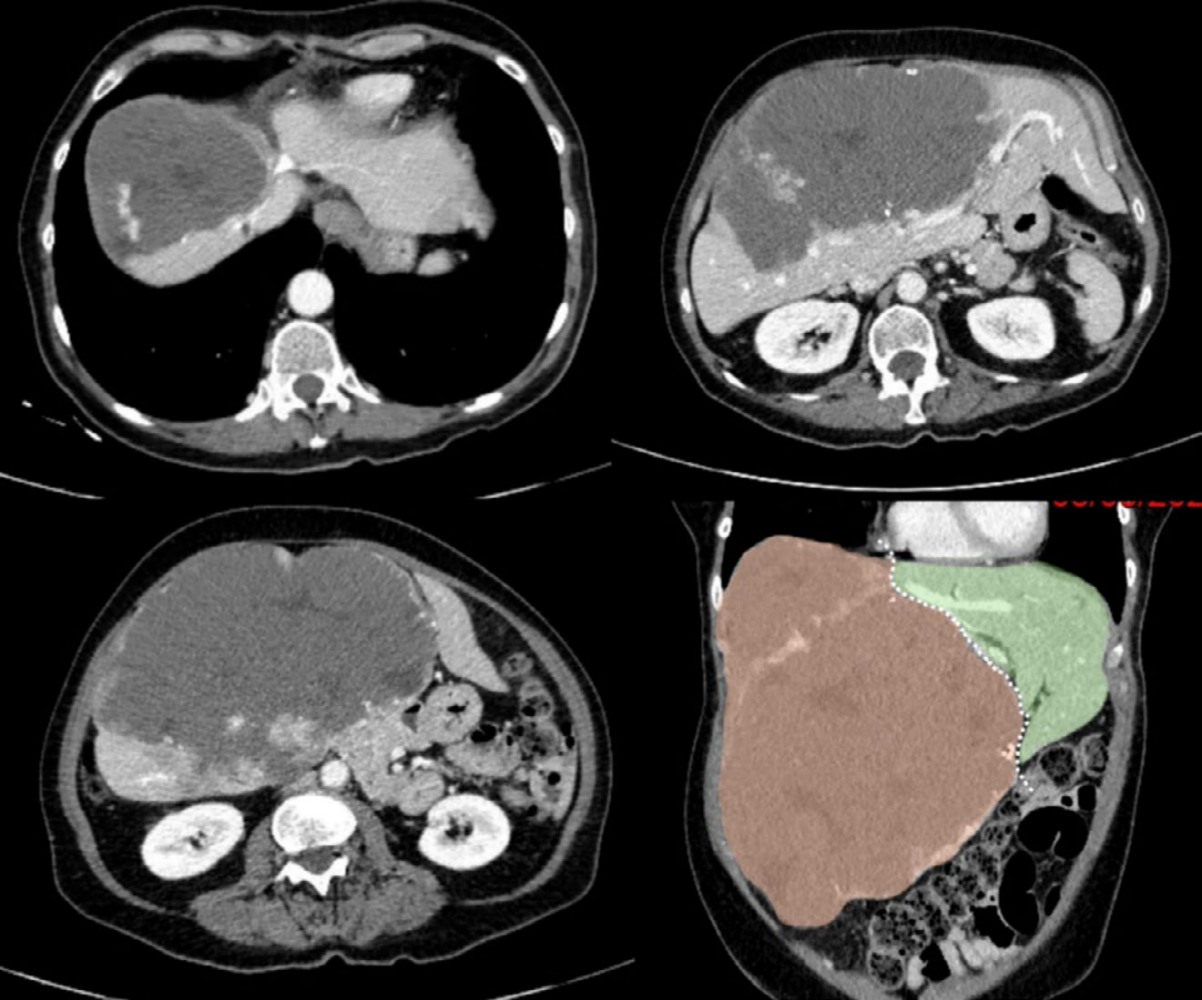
CT scan with giant hemangioma in the right liver. [Color figure can be viewed at wileyonlinelibrary.com]
